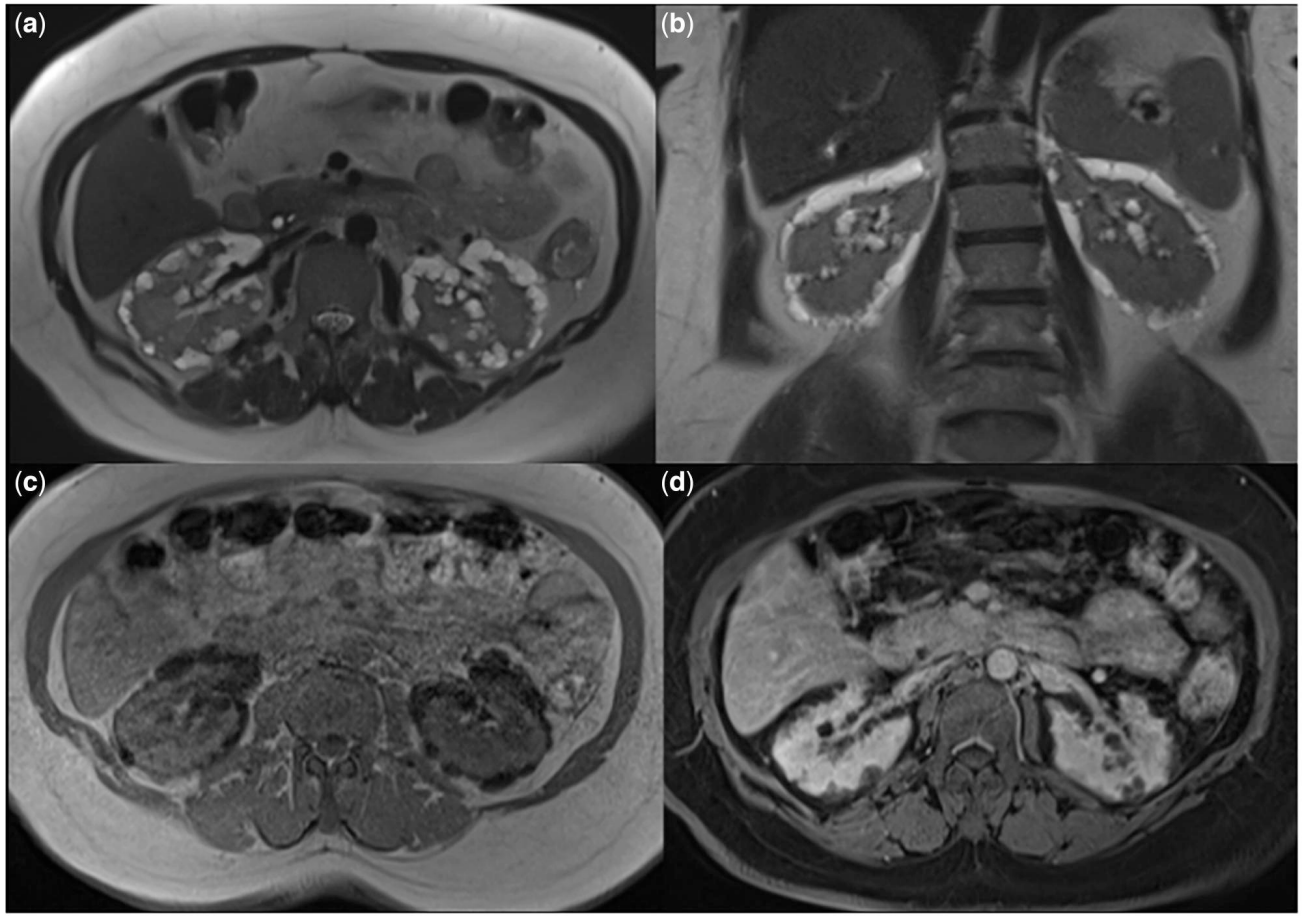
Bilateral perirenal and intrarenal cystic lesions are hyperintense on axial (A) and coronal (B) T2 MR images and hypointense on axial (C) T1 MR images. Contrast enhancement was not seen in bilateral perirenal and intrarenal cystic lesions on axial fat-suppressed contrast enhanced (D) T1 images.
